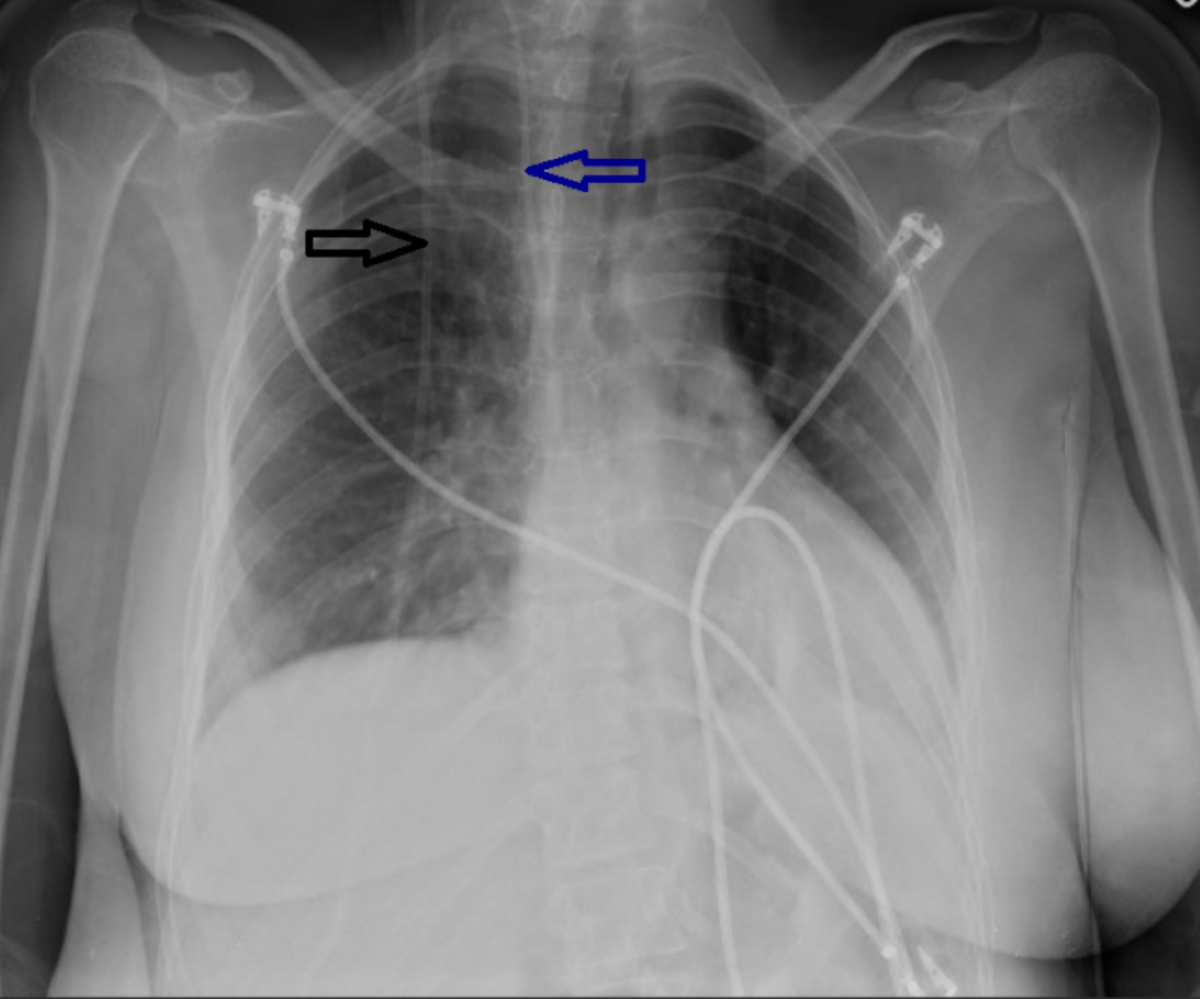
Chest x-ray. Black arrow demonstrates ventriculoperitoneal shunt Blue arrow demonstrates ventriculoatrial shunt
